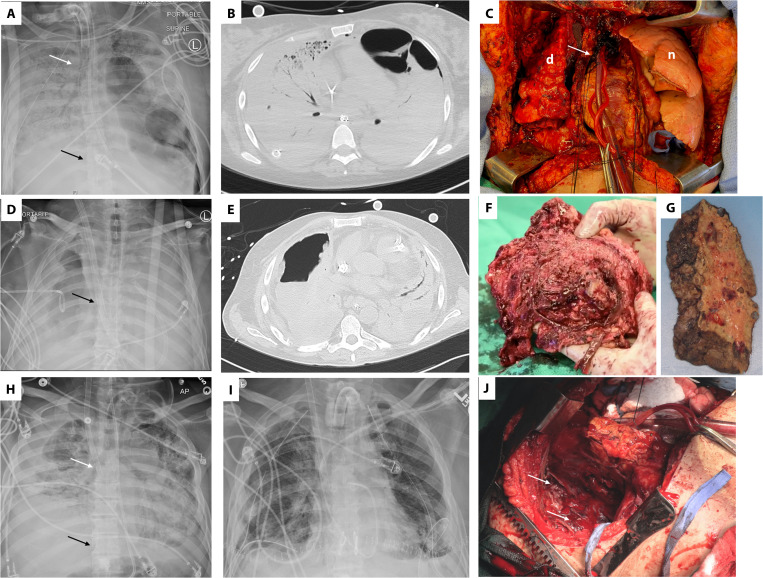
Radiographic and intraoperative findings in lung transplant recipients with severe COVID-19. (A to C) Radiographic and intraoperative findings for case 1 with severe COVID-19 who underwent lung transplantation. (A) Pretransplant chest radiograph (day 38 after onset of ARDS) for case 1, revealing opacification of the right lung and a left lower lobe necrotic cavity attributed to pneumonia caused by *Serratia marcascens*. A tube thoracostomy was required to treat right spontaneous hemothorax and peumothoraces. In addition, the image shows ECMO cannulas (inflow cannula carrying oxygenated blood to the patient is indicated by the white arrow, and outflow cannula carrying deoxygenated blood from the patient to the ECMO machine is indicated by the black arrow). (B) Severe ARDS and lower lung lobe necrosis in case 1 were confirmed by cross-sectional computed tomography imaging. (C) Shown is an intraoperative image revealing contrasting features between the diseased native right lung (d) and the newly transplanted left lung (n). The photograph was taken immediately after the transplant of the left lung and before proceeding onto right lung transplantation. The pericardial sac (white arrow) containing the heart was opened to gain access to the aorta and place the outflow cannula of the venoarterial ECMO. (D to F) Radiographic and intraoperative findings for case 2 with severe COVID-19 who underwent lung transplantation. (D) Pretransplant chest radiograph (at day 98 after onset of ARDS) for case 2 revealing bilateral lung opacifications and a necrotic cavity in the right lung attributed to pneumonia caused by *Pseudomonas aeruginosa*. A chest tube to treat bronchopleural fistula is visible. The dual lumen ECMO cannula is indicated by the black arrow. (E) Computed tomography imaging indicates severe ARDS and development of a necrotic cavity in the right lung. (F) Freshly explanted right lung of case 2 with extensive pleural inflammation and loss of identifiable anatomical planes. (G) Formalin-fixed explanted right lung of case 1 demonstrating the development of lung cavities. (H to J) Radiographic and intraoperative findings for case 3 with severe COVID-19 who underwent lung transplantation. (H) Pretransplant chest radiograph (day 86 after onset of severe ARDS) for case 3, revealing extensive consolidation (lack of air) with right fibrothorax. In addition, the image shows ECMO cannulas (inflow cannula carrying oxygenated blood to the patient is indicated by the white arrow, and the outflow cannula carrying deoxygenated blood from the patient to the ECMO machine is indicated by the black arrow). (I) Posttransplant day 1 chest radiograph for case 3 demonstrating the expected appearance of new lung allografts after bilateral lung transplantation. (J) Intraoperative photograph after implantation of the left lung and explantation of the right lung revealing the right hemithorax. Diffuse pleuritis with severe thickening of parietal pleura and neoangiogenesis (white arrows) were noted as in the other two cases.
